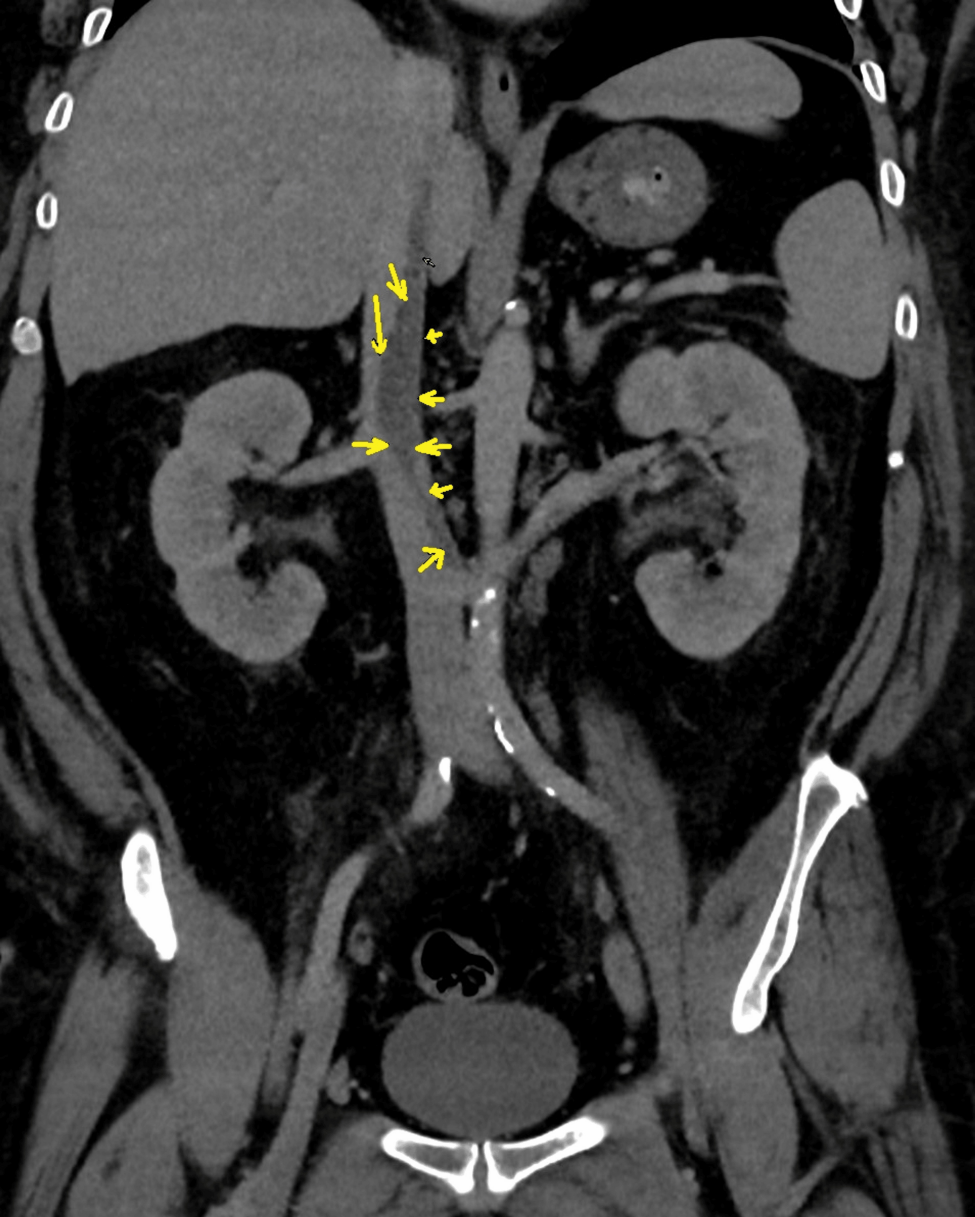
CT showing a long irregular eccentric filling defect (yellow arrow) in the inferior vena cava.
